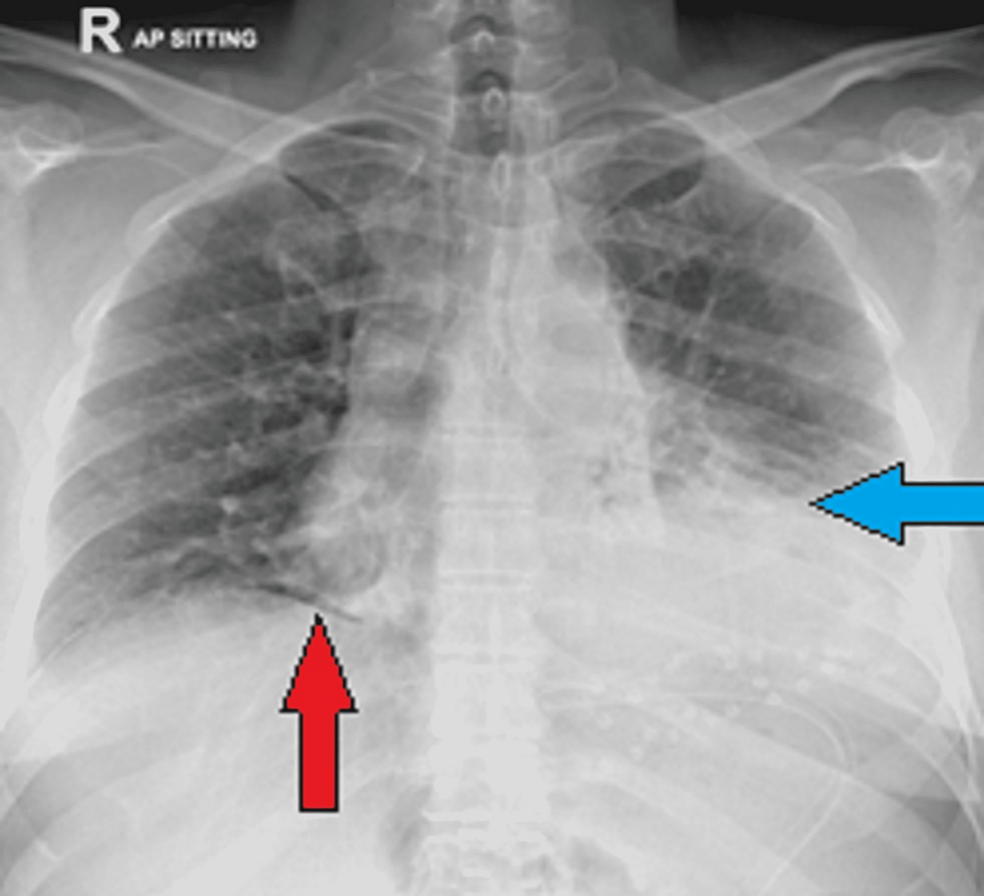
Postoperative X-ray chest showing left lower zone atelectatic changes Traces of post-surgical pneumoperitoneum are seen below the right hemidiaphragm
